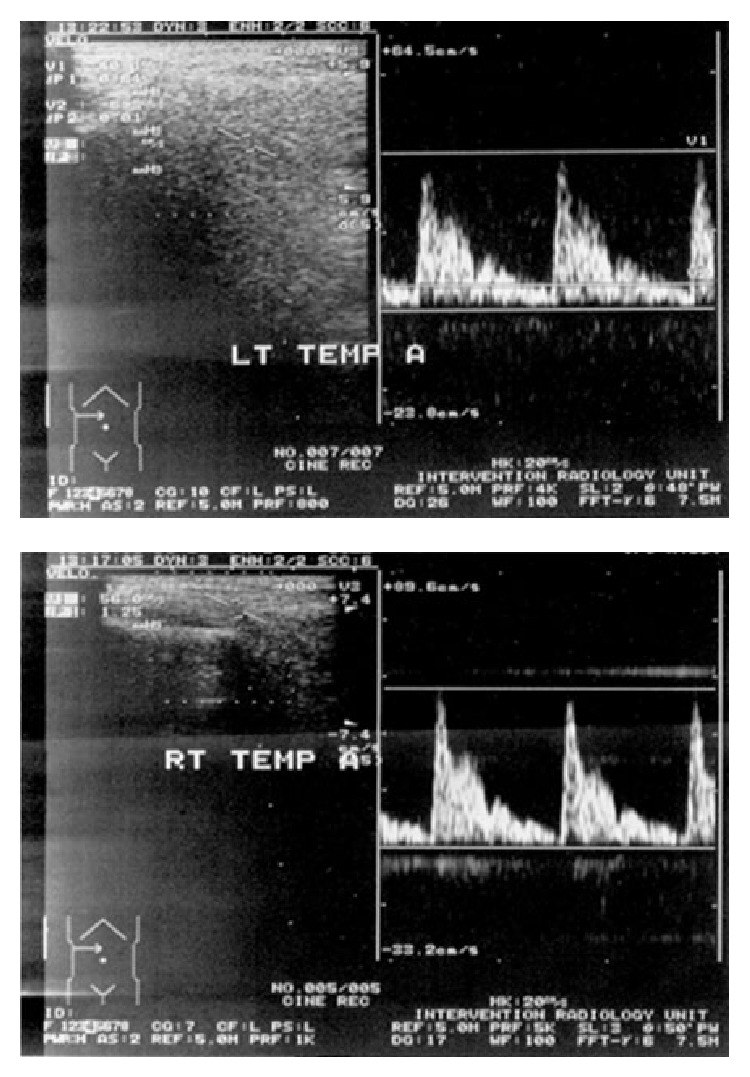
Duplex ultrasound of the temporal arteries. The diameter of the right temporal artery (RT TEMP A) was narrowed compared to the left (LT TEMP A). The resistive index was equal on both.
